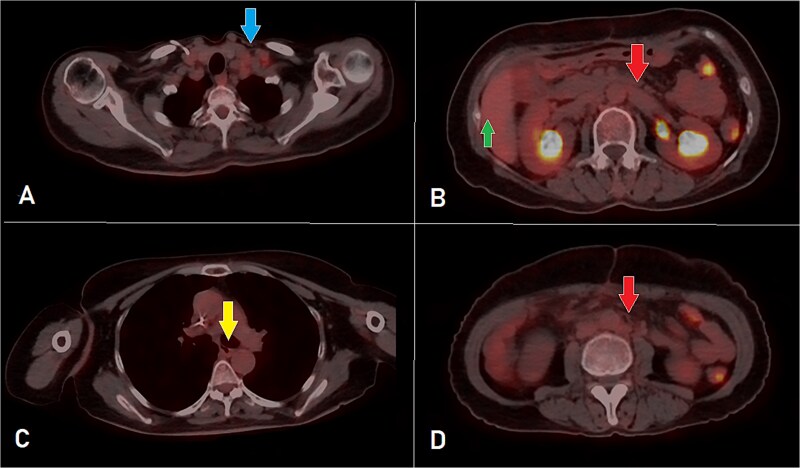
PET-CT scan at the corresponding cuts to Fig. 1 showing great metabolic and morphological regression regarding the previously seen (A) supraclavicular lymph nodes (arrow); (B) retroperitoneal lymph nodes (inferiorly pointing arrow) and hepatic lesion (superiorly pointing arrow); (C) mediastinal (para-oesophageal – station VIII) lymph node (arrow); and (D) retroperitoneal lymph nodes (arrow).
